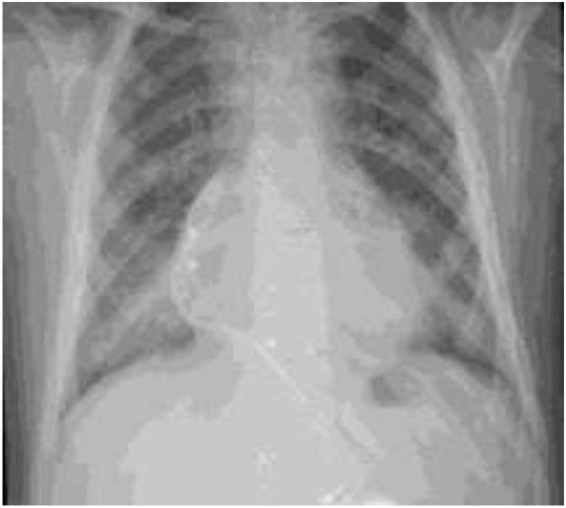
Chest x-ray of the second patient.
